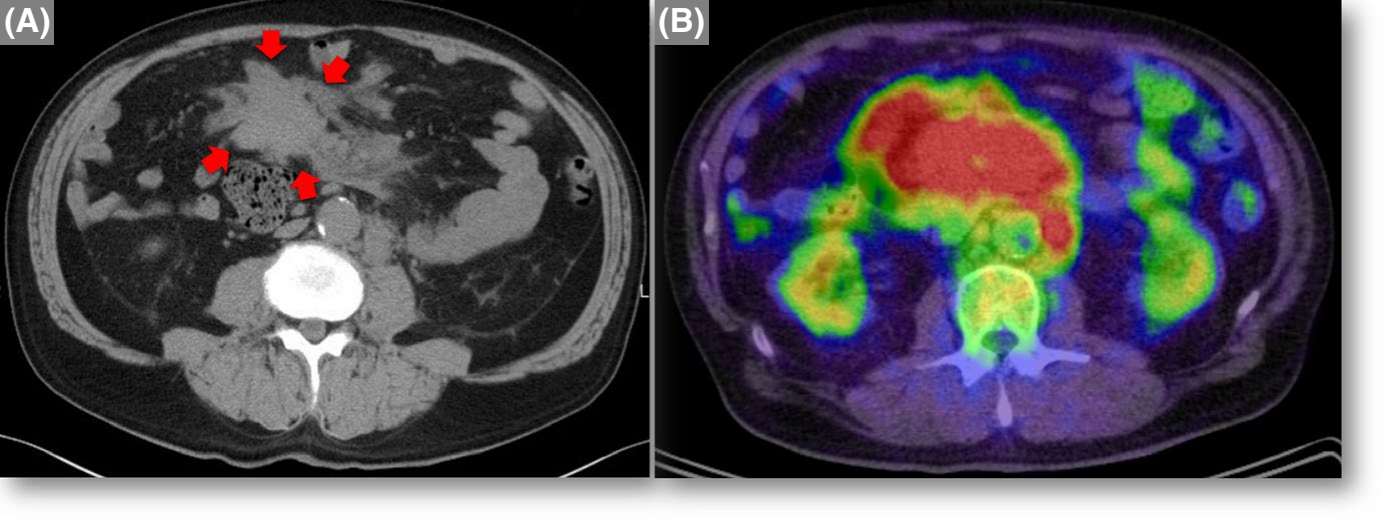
A, Noncontrast CT demonstrated a huge intraperitoneal mass (13 cm diameter) (arrows). B, Fluorine‐18‐fluorodeoxyglucose positron emission tomography‐CT showed a bulky mass with a maximum standardized uptake value of 13.26
